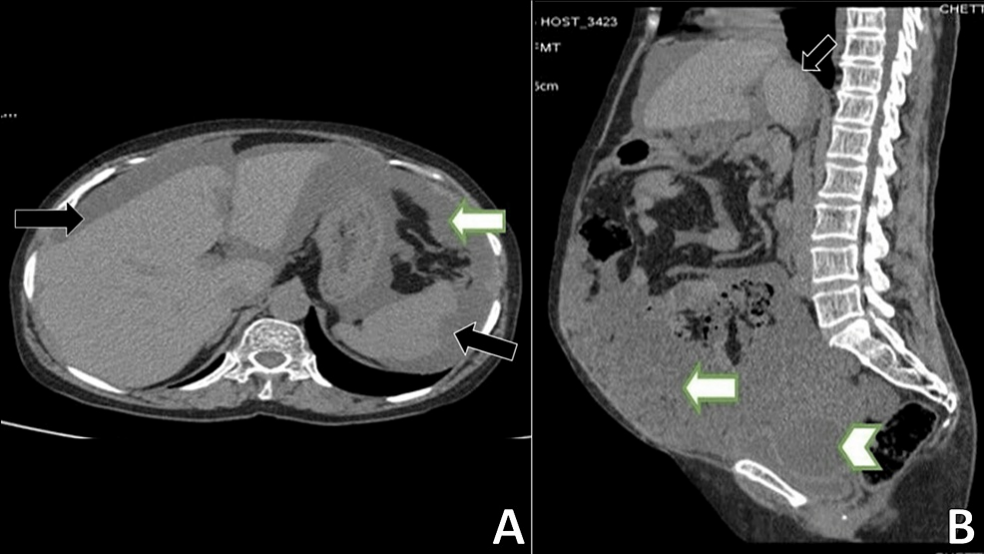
CT abdomen and pelvis: (A) axial view and (B) sagittal view Mucinous appearing free fluid in peritoneal cavity (white arrow) and scalloping of liver and spleen (black arrow) (A). CT abdomen and pelvis showed (B) a complex cystic mass with multiple septations in the pelvis posterior to the bladder causing indentations on the bladder contour (arrowhead). There was mucinous appearing free fluid in the peritoneal cavity (white arrow).
